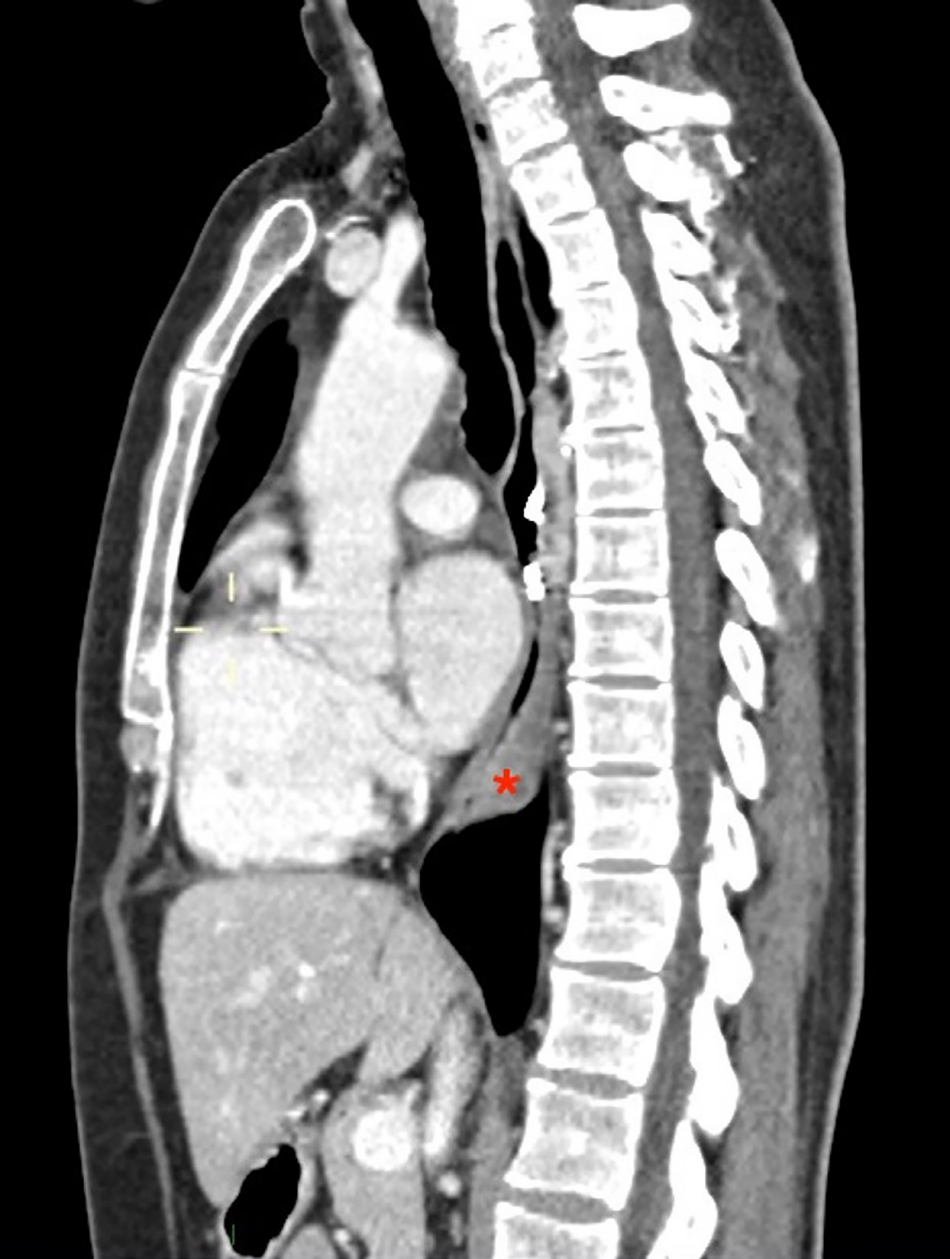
Contrast-enhanced, sagittal plane CT scan of the chest performed 1 month after POEM, showing a marked reduction of the dimensions of the submucosal esophageal haematoma. The patency of the esophageal lumen is now restored.
